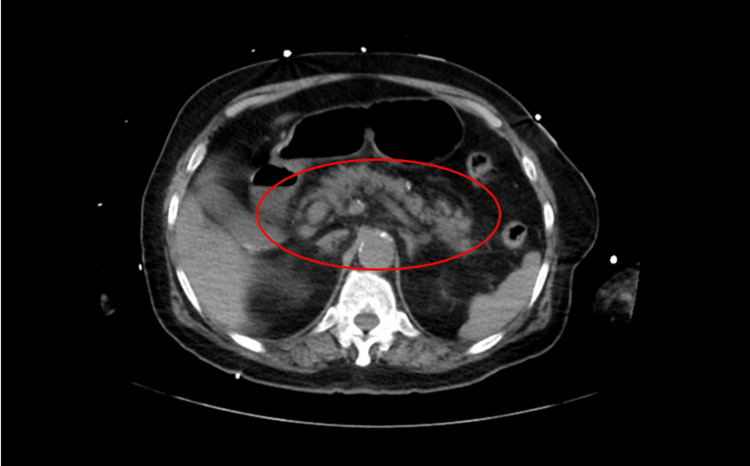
Computed tomography of the abdomen without contrast showing peripancreatic fat stranding on admission
